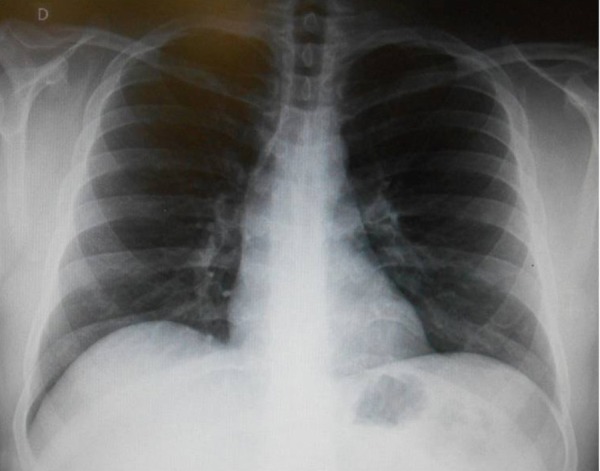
Chest radiograph shows normal heart area and pulmonary vascular bed. The
